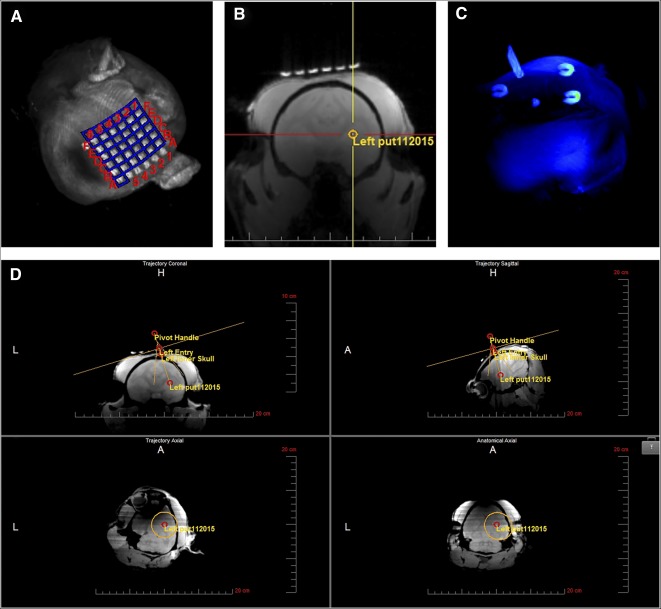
Trajectory alignment. (A): Maximum intensity projection (MIP) of the SmartGrid, a 6 × 6 array of magnetic resonance imaging (MRI)‐sensitive gadolinium‐filled squares placed on the skull covering the estimated entry point of the needle. (B): Three‐dimensional T1‐weighted gradient echo series transferred to the ClearPoint workstation and used to select the target. (C): MIP of the SmartFrame trajectory guide showing fiducial markers used by the software to segment the SmartFrame and to calculate magnetic resonance scanning parameters for planning the trajectory of the cannula. (D): Point of entry and trajectory to the target set based on the anterior and posterior commissures and the midsagittal plane and adjusted, if needed, to ovoid particular structures, ventricular systems, or blood vessels using the “fly‐through” trajectory option. Abbreviations: A, anterior; H, head; L, left.
